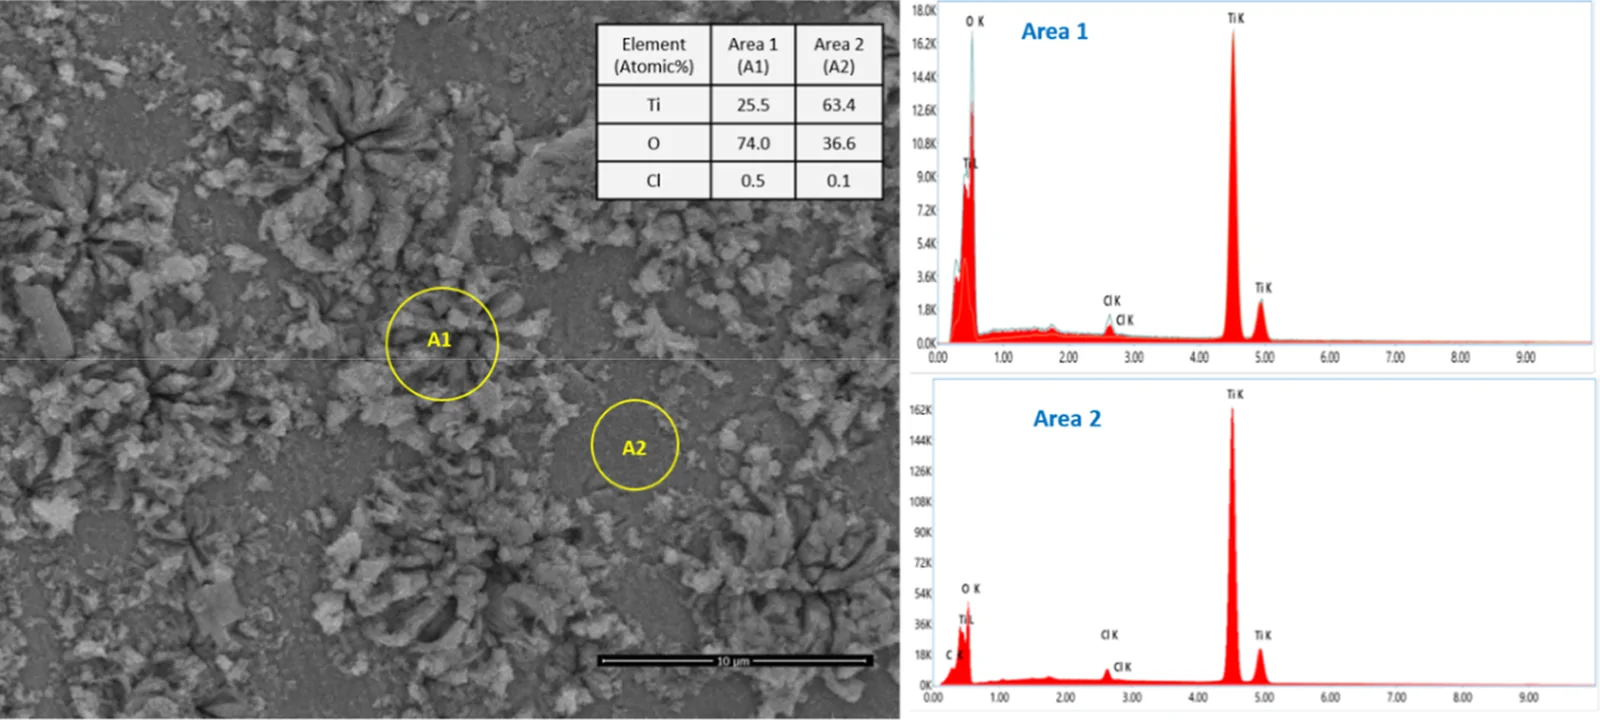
Energy dispersive X-ray analysis (EDAX) of two demarcated
